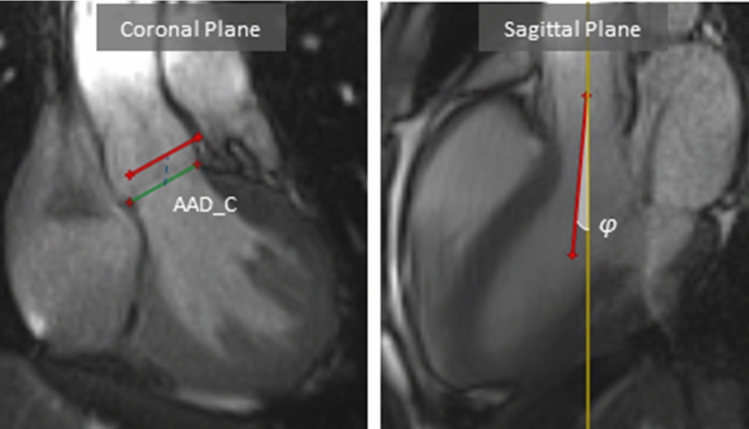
Motion of the aortic root in 2 planes. (left) Coronal cine-MR images showing the position of the ventriculo-aortic junction (VAJ) in systole (green line) and in diastole (red line). The blue dotted line represents the maximal aortic annulus displacement in the coronal plane (AAD_C) linking the mid-points of the VAJ in systole and diastole. (right) Sagittal cine-MRI images depicting the motion vector in the sagittal plane (red arrow) and the cross-reference line (yellow line). The angle, *φ*, between the red vector and yellow line represents the angle of rotation between the two planes. The total displacement from diastole to systole was calculated as AAD_C*cos(*φ*) and was found equal to 9.5 mm
